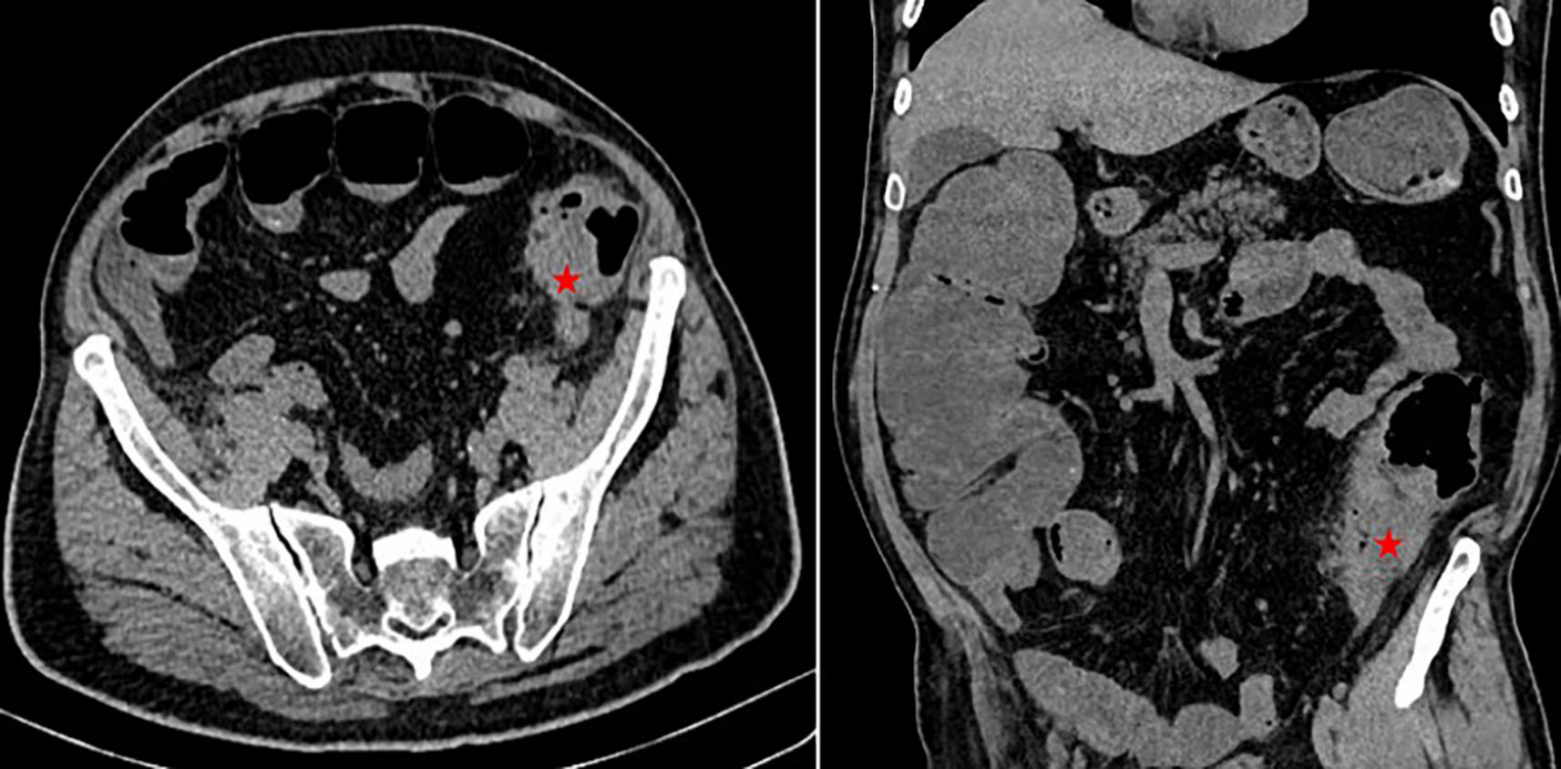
Abdominal CT scan showing acute intestinal obstruction with an obstructive tissular mass at the sigmoid colon (red asterixis).
